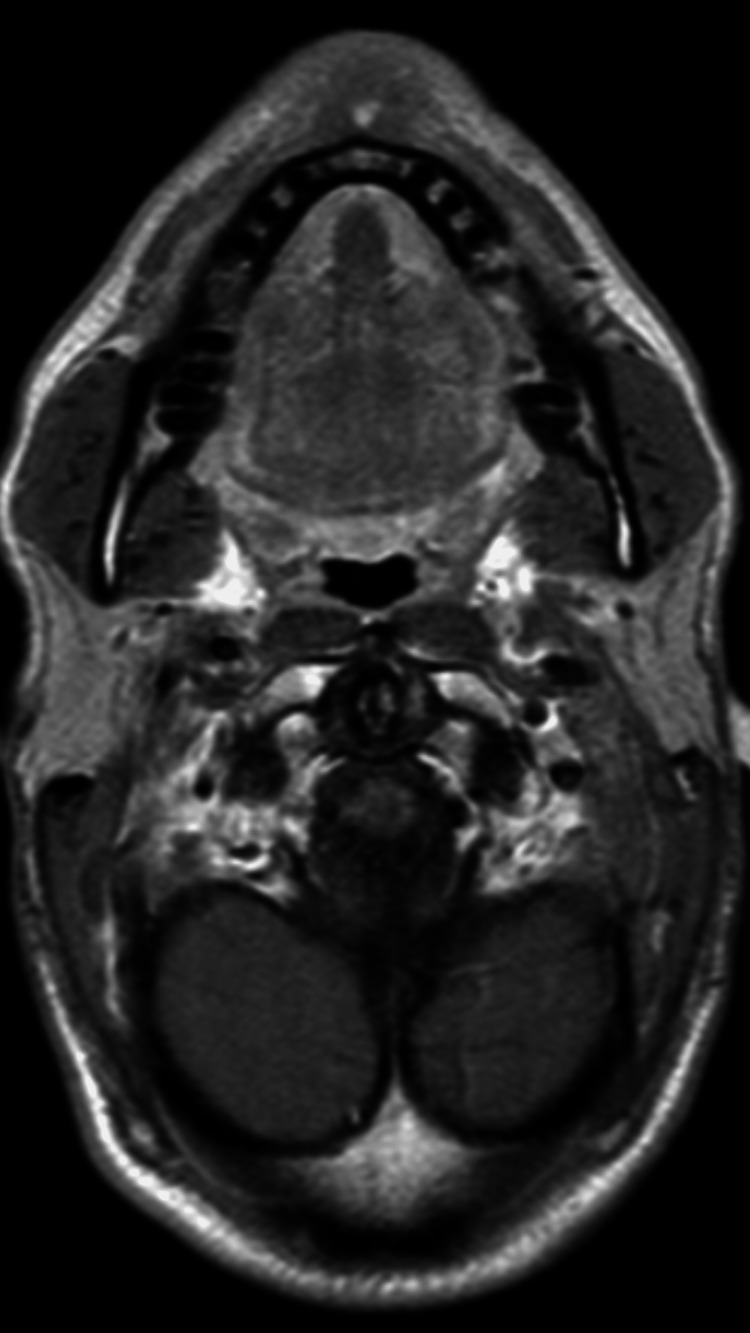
Axial MRI T1w showing no abnormalities arising from the left parotid gland or parapharyngeal space (PPS)
